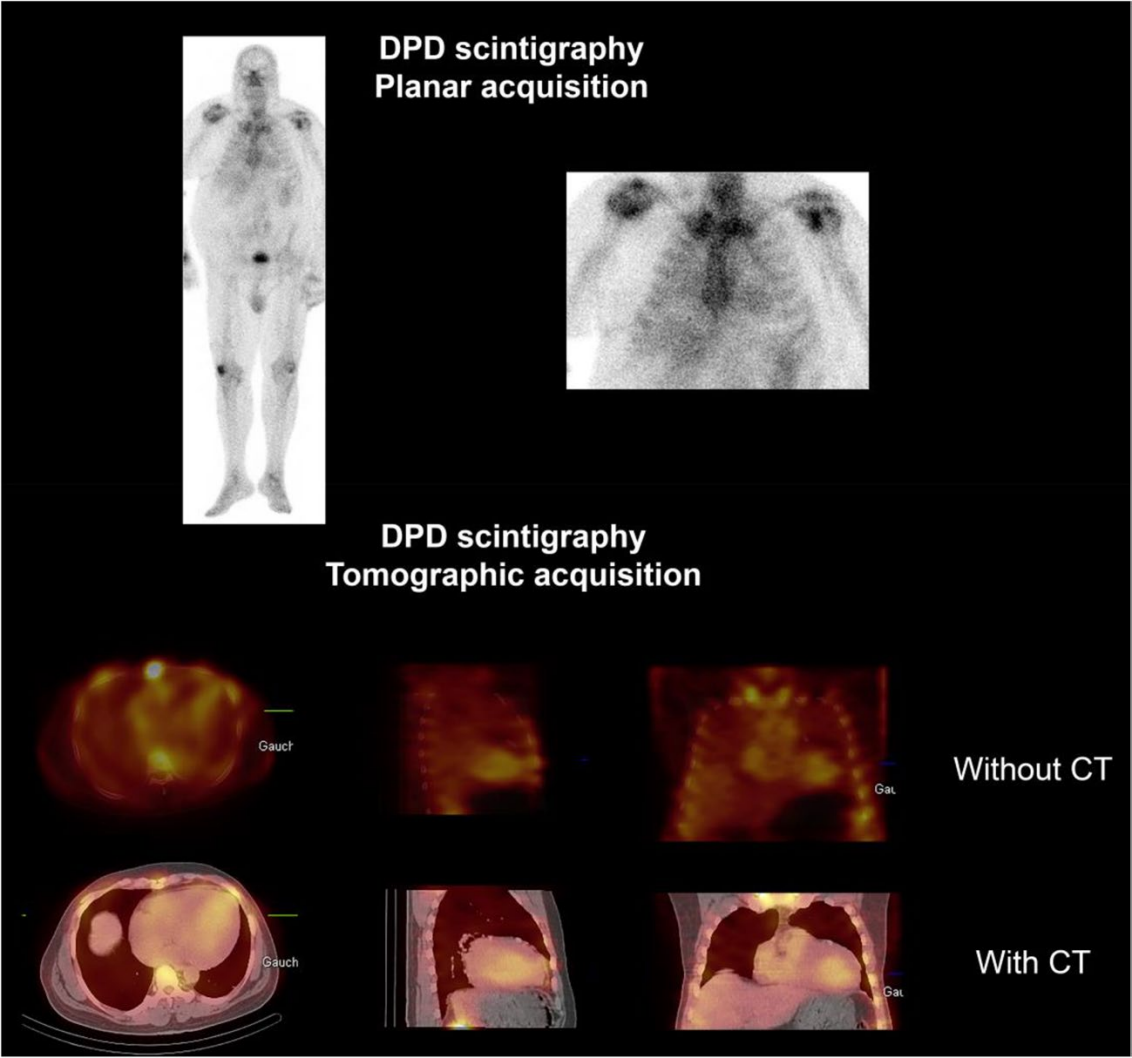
False-positive cardiac uptake using bone scintigraphy with blood pool residual activity. Planar acquisition was scored with grade 1 according to Perugini classification. SPECT acquisition fused with low-dose CT shows that the signal is located in left ventricular cavity, without bone tracer uptake within the myocardium
